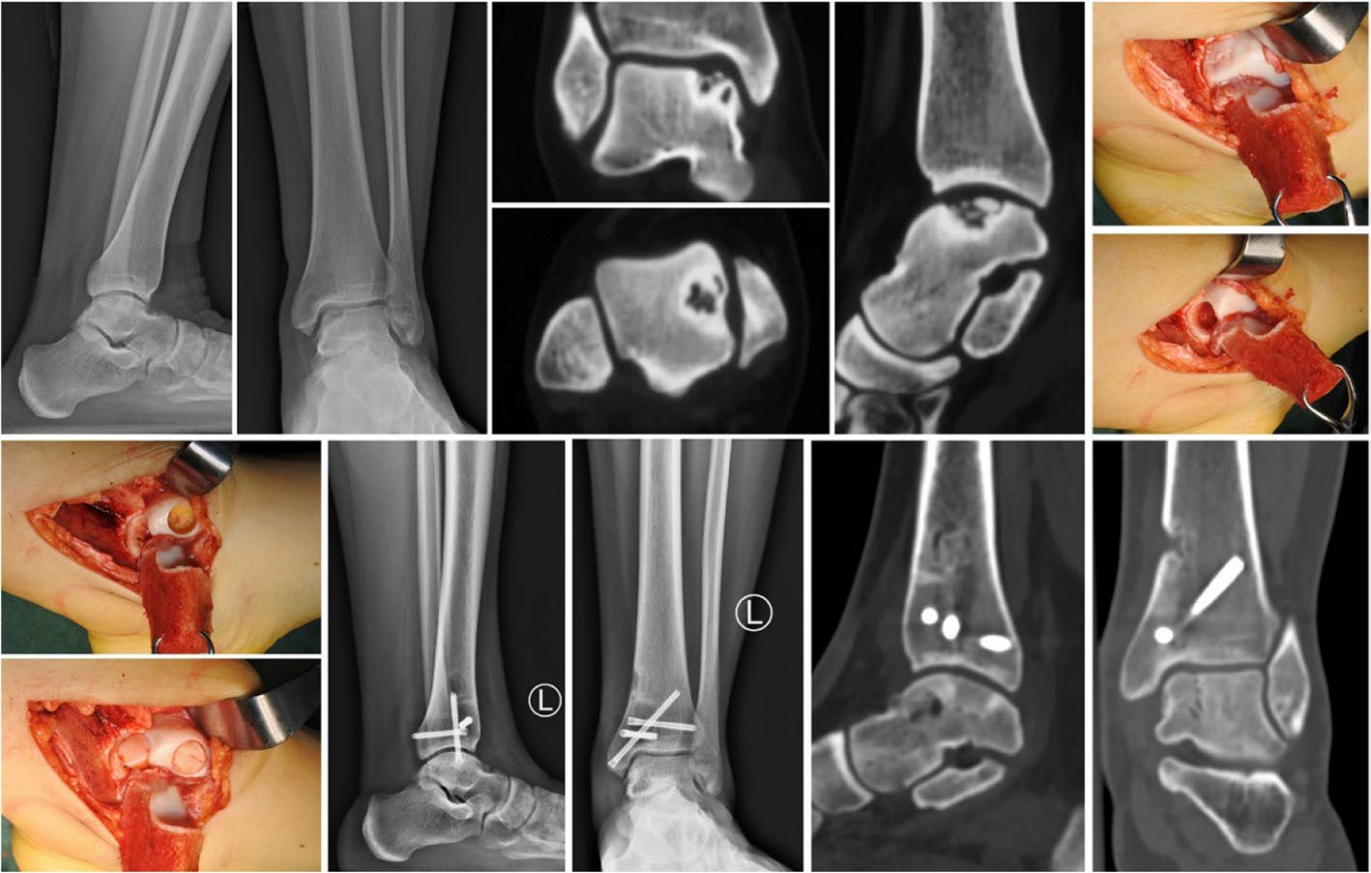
Case1, female, 31y, osteochondral lesions of left talus. A-E: preoperative imaging (X - ray and CT) supported the diagnosis; F-G: the lesion was exposed through the medial malleolus triplane osteotomy and debrided; H-I: AOT for OLTs from a non-weight-bearing area, and the donor defect was filled with a bone graft with periosteum from the tibia; J-M: at 37-month follow-up, imaging showed that the grafts had fused with the original bone in the talus, and the medial malleolus had achieved anatomic union
